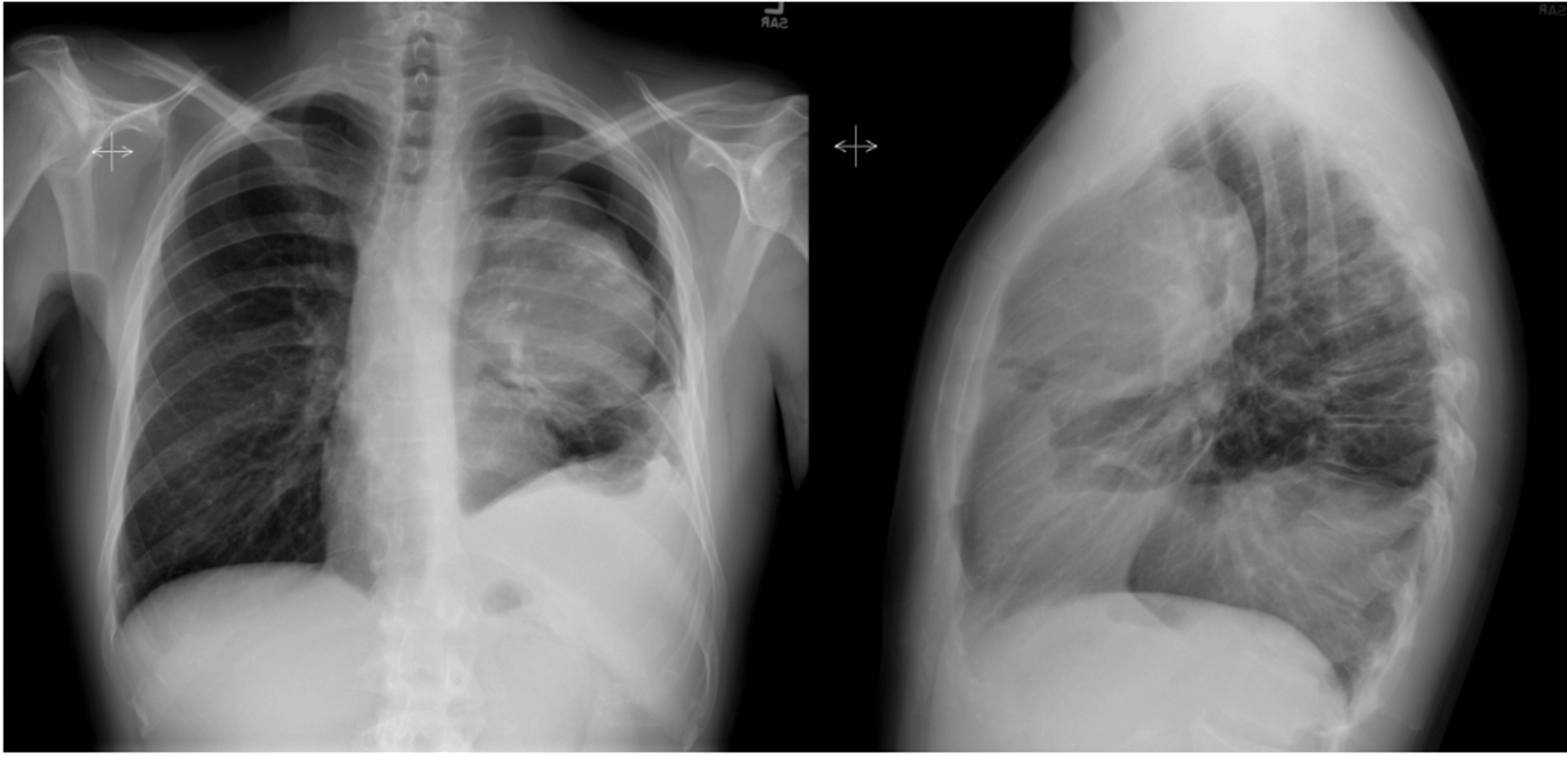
Anteroposterior and lateral chest X-ray four months after initiating treatment with improved aeration of the left lower lobe and lingula. Persistent atelectasis of the left upper lobe is noted.
